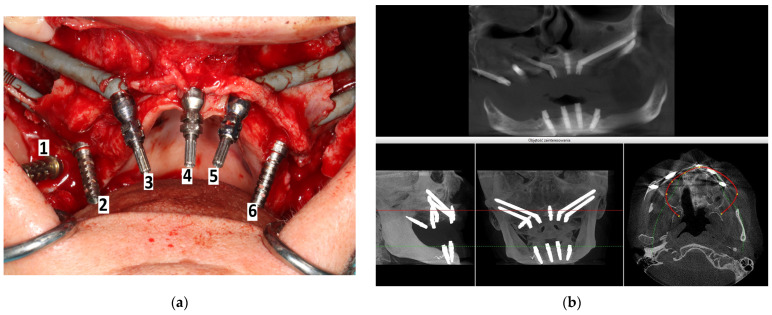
(a) Intrasurgical implant placement legend: 1—pterygoid; 2—intramaxillary and intrasinus; 3—extramaxillary and extrasinus; 4—traditional endosseous; 5—intramaxillary and extrasinus; 6—intramaxillary and intrasinus. (b) The same patient on CBCT taken on the day of surgery.
